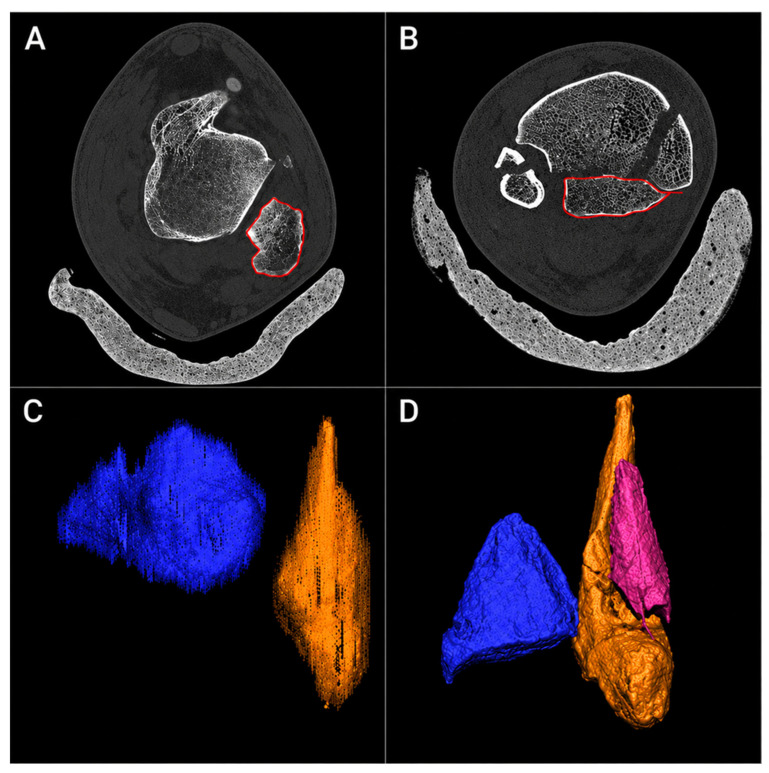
CT-based manual volumetric segmentation and three-dimensional reconstruction of malleolar fracture fragments. (A) Axial thin-slice CT image demonstrating manual contour-based segmentation of the lateral malleolar fragment using a free-hand region of interest. (B) Axial thin-slice CT image demonstrating manual contour-based segmentation of the posterior malleolar fragment. The red outlines in panels A and B indicate the manually drawn contour boundaries used for segmentation. (C) Isolated three-dimensional reconstructed fracture fragments after volumetric segmentation, showing the posterior malleolar fragment in blue and the lateral malleolar fragment in orange. (D) Three-dimensional reconstructed CT fragment model demonstrating a multifragmentary configuration, including the posterior malleolar fragment in blue, the primary lateral malleolar fragment in orange, and a secondary lateral malleolar fragment in pink. These reconstructed models were used to support volumetric assessment and morphological review of the fracture fragments.
